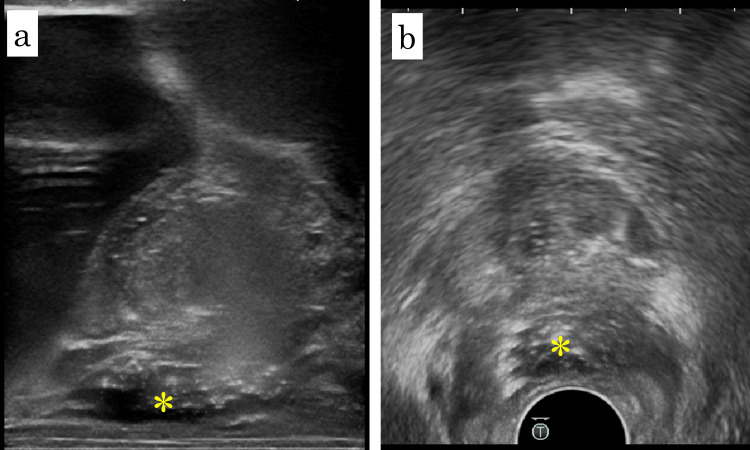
Sagittal and axial TRUS images after spacer placement Sagittal (a) and axial (b) transrectal ultrasound (TRUS) images obtained after hydrogel spacer placement. The hydrogel (yellow asterisk) is visible between the posterior prostate and anterior rectal wall, confirming successful separation.
